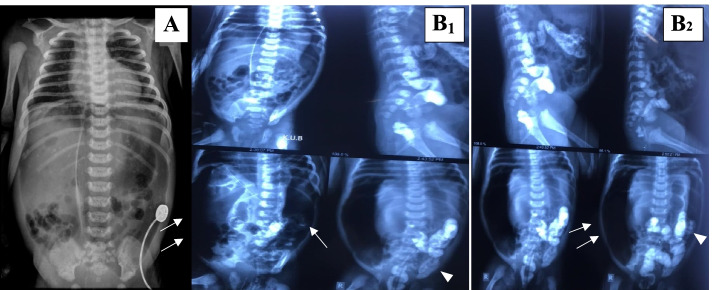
A Plain radiograph showing pneumoperitoneum. B_1_–_2_: water-soluble contrast enema, which indicated leakage of contrast (Arrowheads). Note the extensive pneumoperitoneum in the margins of the image (Arrows)
